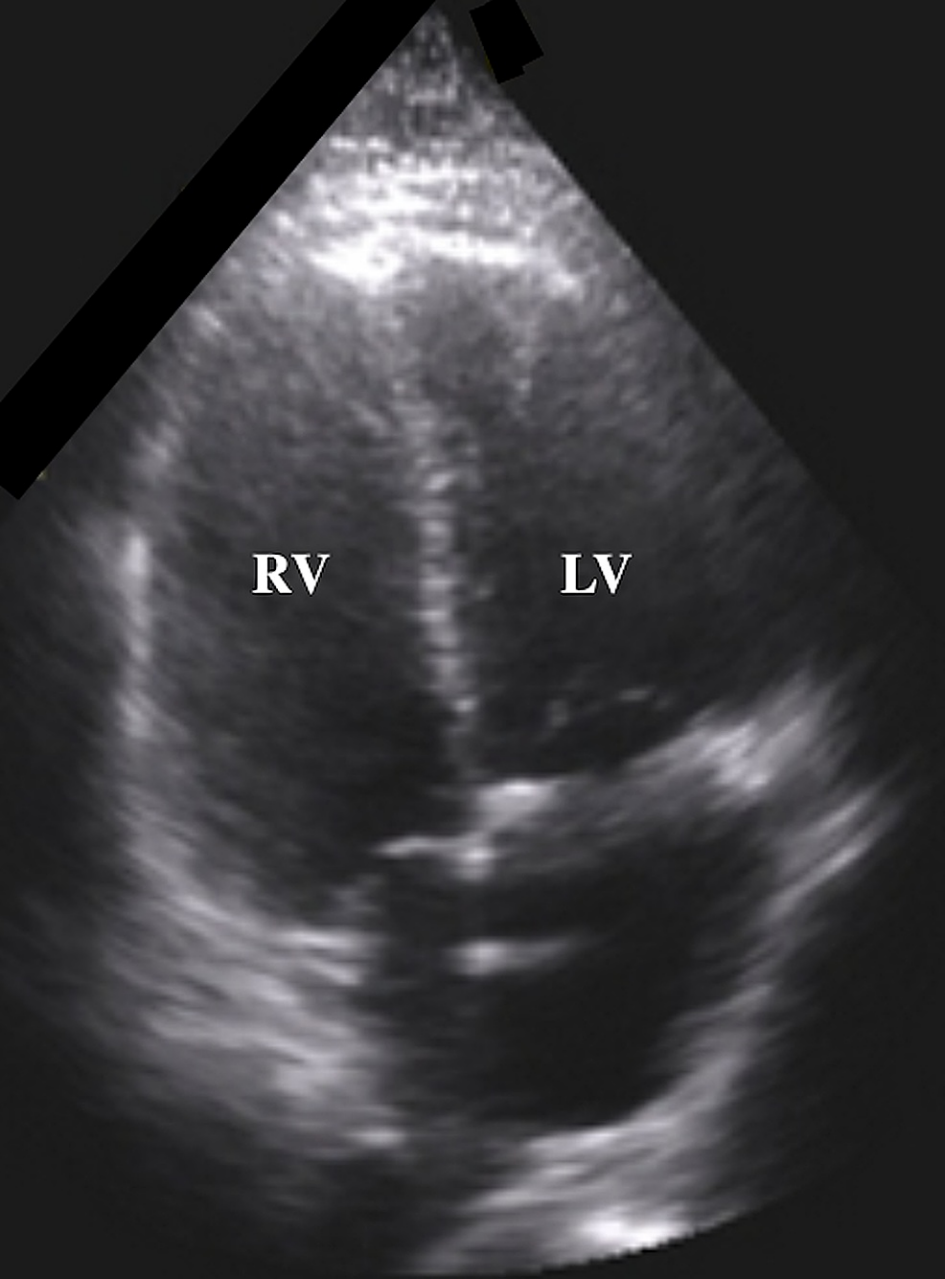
Bedside point of care ultrasound (POCUS) during CPR showed a dilated RV and an RV:LV ratio greater than 1, indicating an increase in RV afterload likely secondary to a PE given the clinical context. CPR - cardiopulmonary resuscitation; RV - right ventricle; LV - left ventricle
